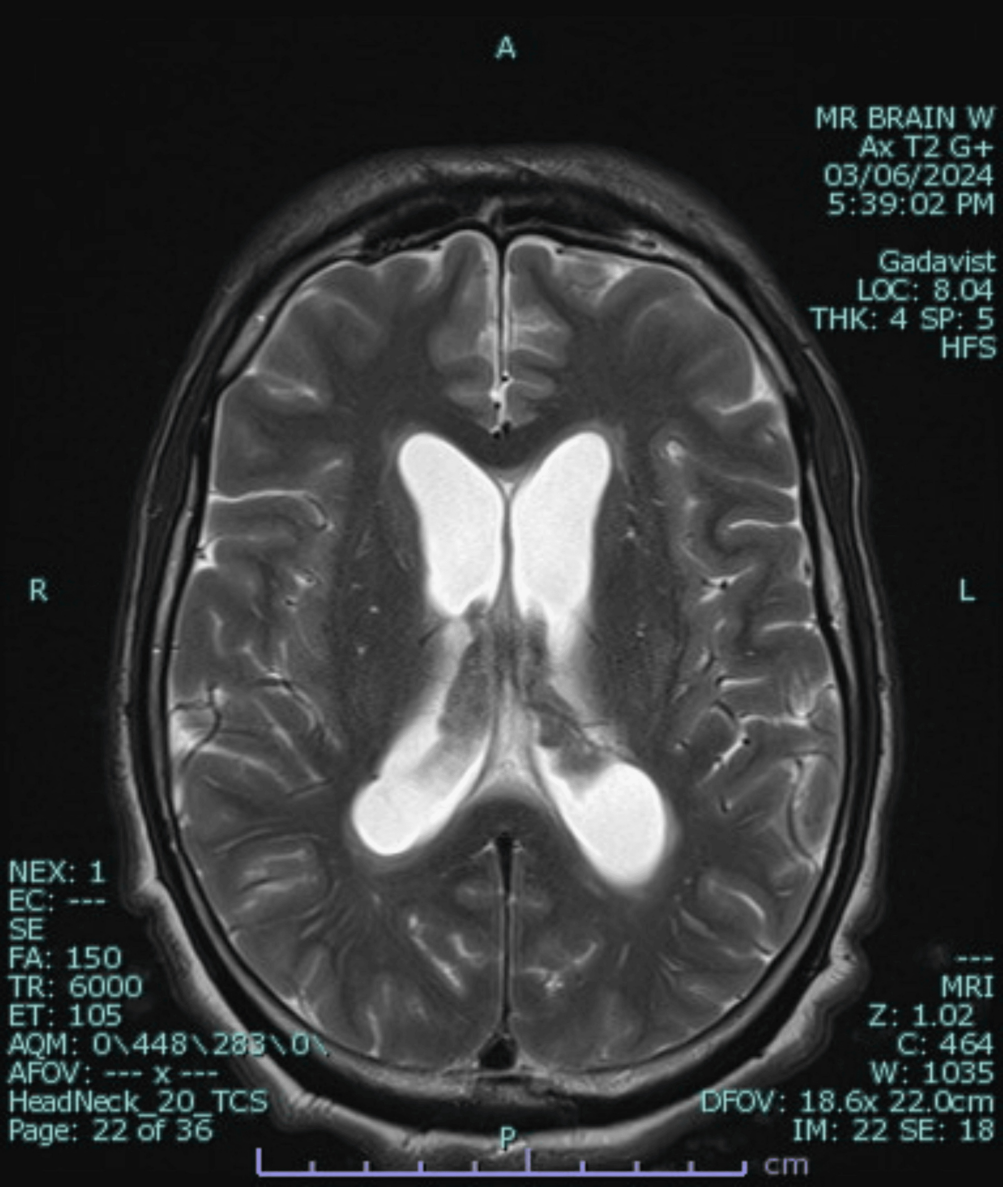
Axial T2-weighted MRI showing prominent bilateral hydrocephalus, choroid plexitis, and periventricular white matter edema
